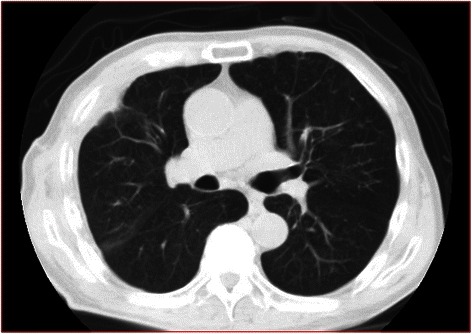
Chest computed tomography image after six months shows right lung expansion and no residual space in the thoracic cavity
